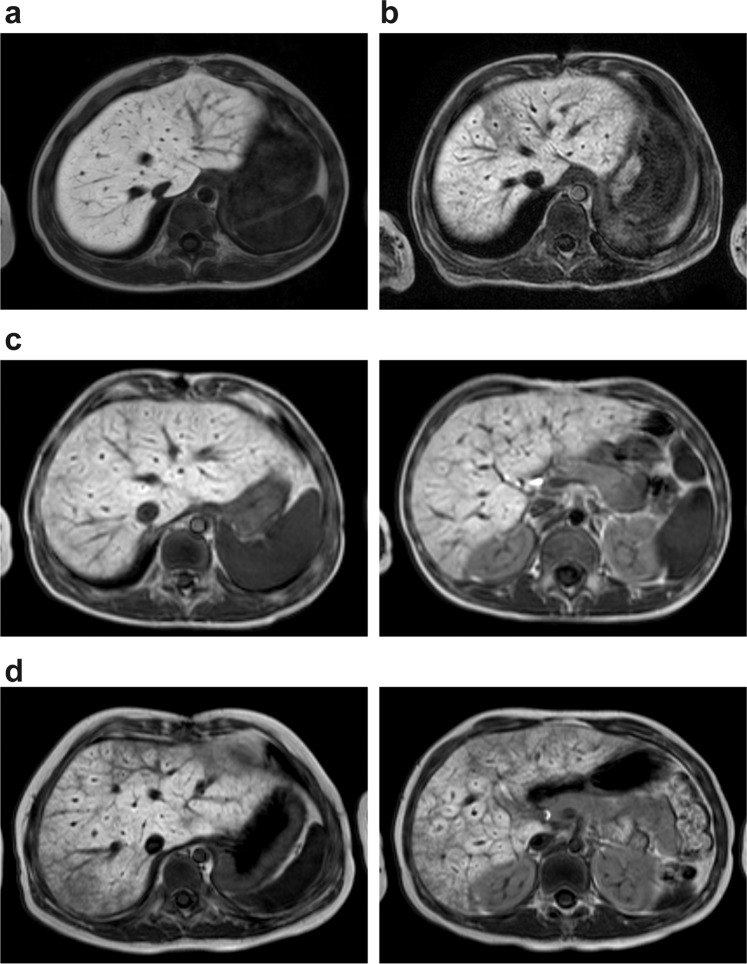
(a) Grade 1: normal liver enhancement on gadolinium ethoxybenzyl diethylenetriaminepentaacetic acid magnetic resonance imaging (EOB-MRI). Normal enhancement shows homogeneous enhancement of the liver parenchyma with high signal intensity, a sharp liver periphery, and no dilation of the hepatic veins. (b) Grade 2: segmental decreased enhancement of the liver parenchyma around the hepatic vein perfusion area, which indicates mild poor enhancement. (c) Grade 3: lobar or diffuse decrease in enhancement on four slices or fewer. Both images are from the same patient. The left panel shows mild diffuse decrease in enhancement, whereas the right panel shows normal enhancement in a different section. (d) Grade 4: diffuse decreased enhancement of the liver. Mosaic enhancement of the liver parenchyma involves the hepatic vein perfusion area with a specific enhancement pattern characterised by a heterogeneous “frog spawn-like” enhancement. The panels correspond to different sections from the same patient and show diffuse poor enhancement.
